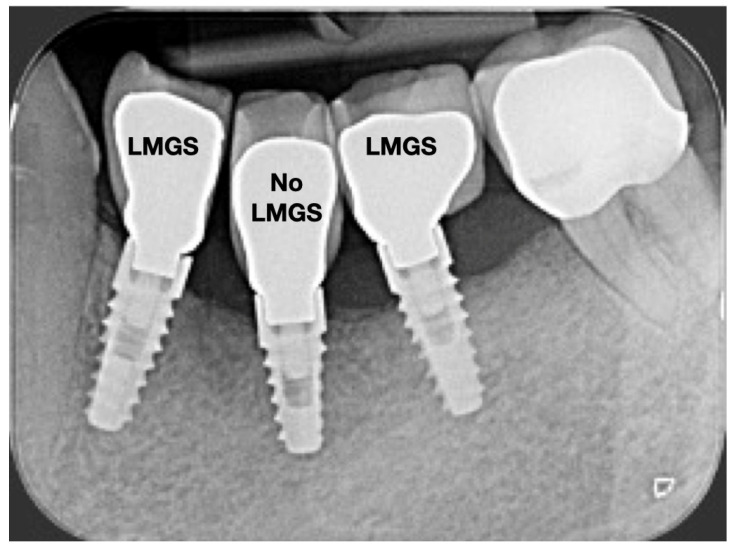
Radiograph of the same three (3) implants at 5 years. (LMGS = with a laser-microgrooved collar surface; No-LMGS = without a laser-microgrooved collar surface).
